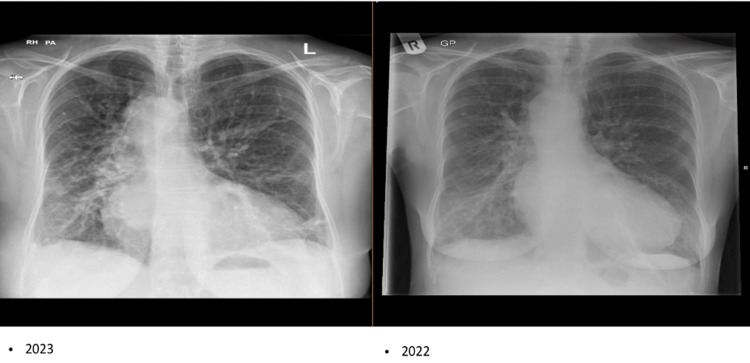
The chest X-ray only revealed some signs of fluid overload in the image on the left. Increased cardiothoracic ratio (CTR). Presence of Kerley b lines. Upper lobe diversion and right-sided pleural effusion with bluntness of costophrenic angles
